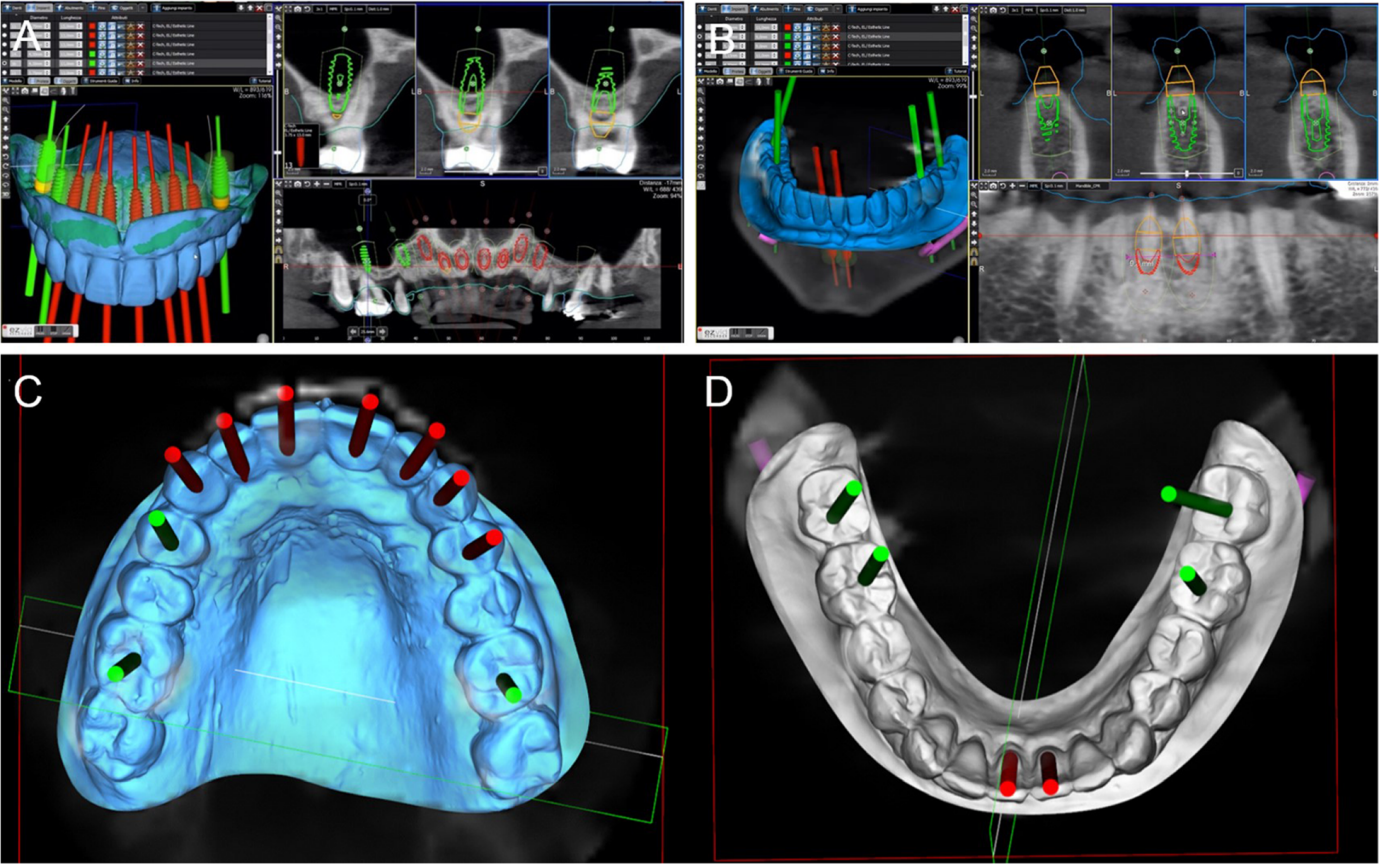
Prosthetically driven 3D implant planning. a In a guided surgery software (RealGuide®, 3Diemme, Como, Italy) the. STL file with the ideal teeth morphology (model of the maxilla with included wax-up) was imported and superimposed on the bone anatomy, obtained from the CBCT. Ten immediate post-extraction implants (#16, #14, #13, #12, #11, #21, #22, #23, #24, #26) were planned in the cross sections of the CBCT (placement of #16 and #26 required maxillary sinus augmentation). The implant position, inclination and depth were carefully planned, trying to engage the fixtures as much as possible into the bone, to increase primary implant stability in the fresh extraction sockets, and taking into account the emergence profile and the overlying future prosthesis, so that implants were placed in a prosthetically driven matter. The final prosthetic plan foresaw rehabilitation with single crowns in the frontal area (from #14 to #24) and rehabilitation with partial fixed prosthesis for the posterior sectors (from #15 to #17, and from #25 to #27, respectively). b A similar procedure was performed in the mandible, with the superimposition of the. STL file with the ideal teeth morphology on the bone, and therefore the implant planning. Six implants were originally planned (#47, #46, #41, #31, #35, #37) in the cross sections of the CBCT. Once again, the implant position, inclination and depth were carefully planned, trying to engage the fixtures as much as possible into the bone, to increase primary implant stability, and taking into account the emergence profile and the overlying future prosthesis, so that implants were placed in a prosthetically driven position. c, d Visualization of the implant emergence profiles in relation to the ideal position of the teeth and the prosthetic plan, in the maxilla and mandible. The prosthetic axes of the posterior implants emerged in the masticatory center of each tooth, in the anterior individual zirconia abutments cemented on titanium bases were chosen. On the upper jaw ten implants were planned, on the lower jaw six
